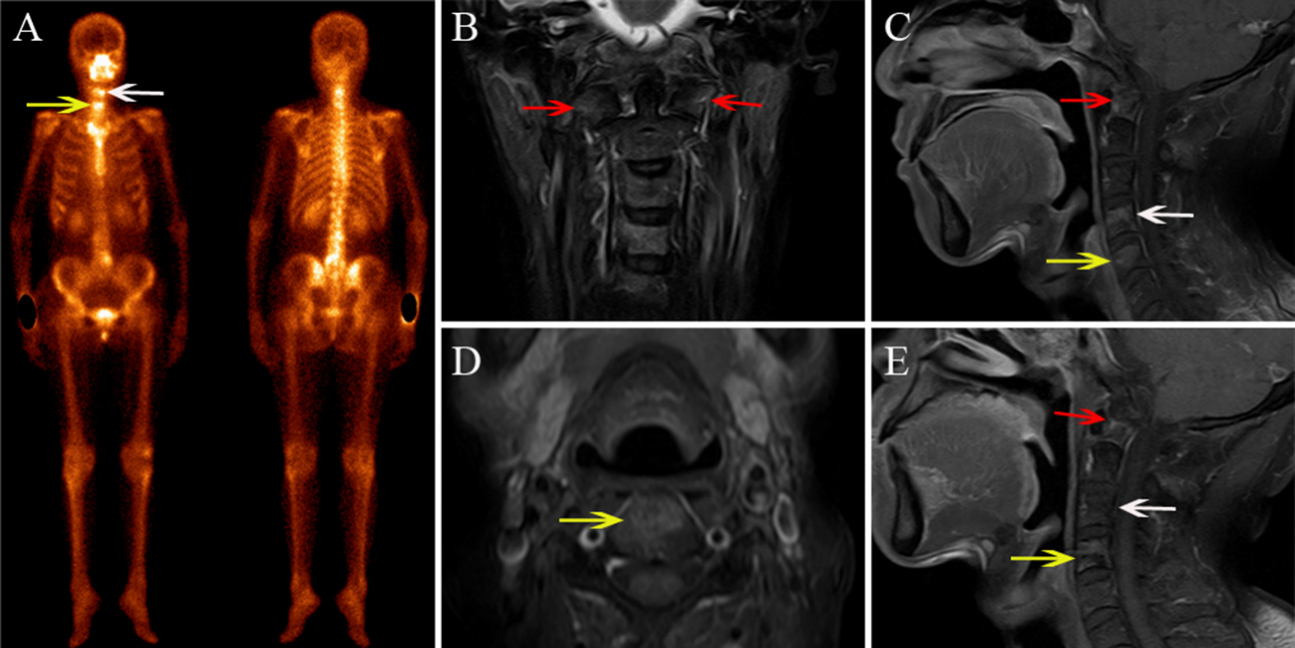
A 48-year-old male diagnosed with cervical spine ORN after RT for NPC. (A) BS shows increased radiotracer uptake in C4 (white arrow) and C5 (yellow arrow). (B) Coronal FS T2-weighted image shows hyperintensity in the bilateral aspect of C1 (red arrow), C4, and C5. (C,D) Sagittal enhanced T1-weighted image (C) and axial enhanced T1-weighted image (D) show mild enhancement in the lesions. (E) MRI follow-up after 7 months; the sagittal contrast-enhanced T1-weighted image shows that the area of the lesions has shrunken and the enhancement has declined in C4 and C5, while the signal change of C1 has remained stationary.
